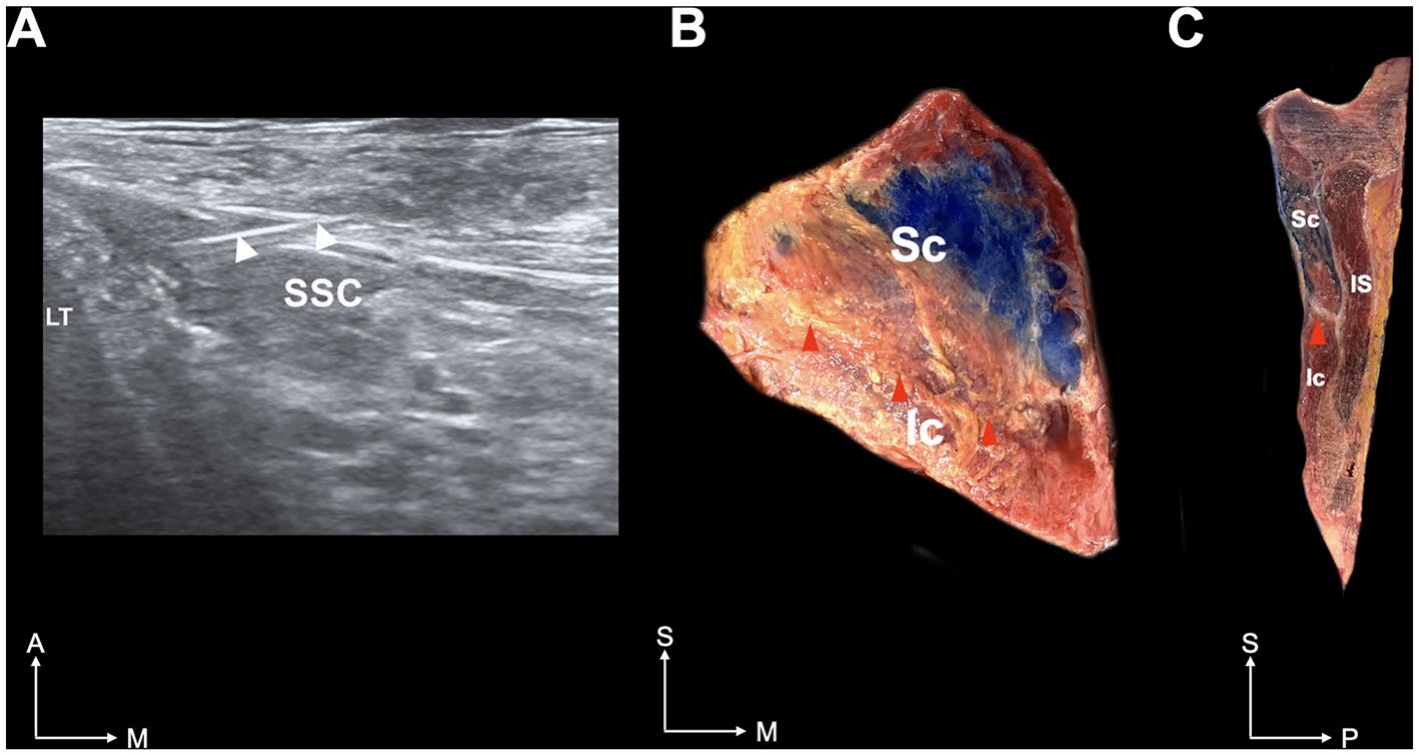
Ultrasound-guided image (A) of an injection into the subscapularis muscle (B) and sectional image (C) of the corresponding injected site. The ultrasound image demonstrates the needle placement (arrowheads). The blue dye is targeted to the superior compartment. The compartments are shown to be separated by the fascial septum. Red dotted line and red arrowhead indicate the fascial septum. *LT* lesser tubercle, *SSC* subscapularis muscle, *Sc* superior compartment of the SSC, *Ic* inferior compartment of the SSC; *IS* infraspinatus, *A* anterior, *M* medial, *S* superior, *P* posterior.
